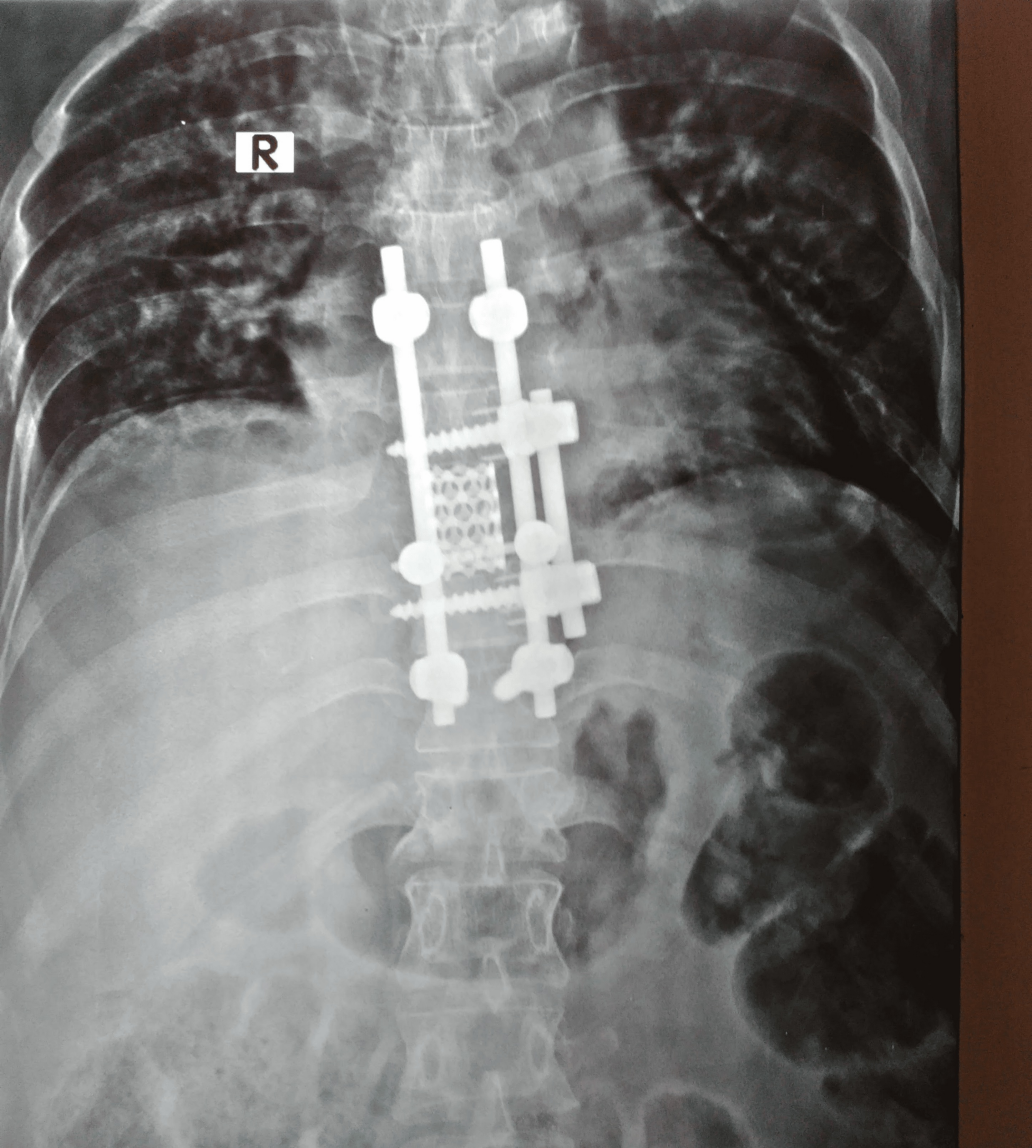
Postoperative X-ray AP view
